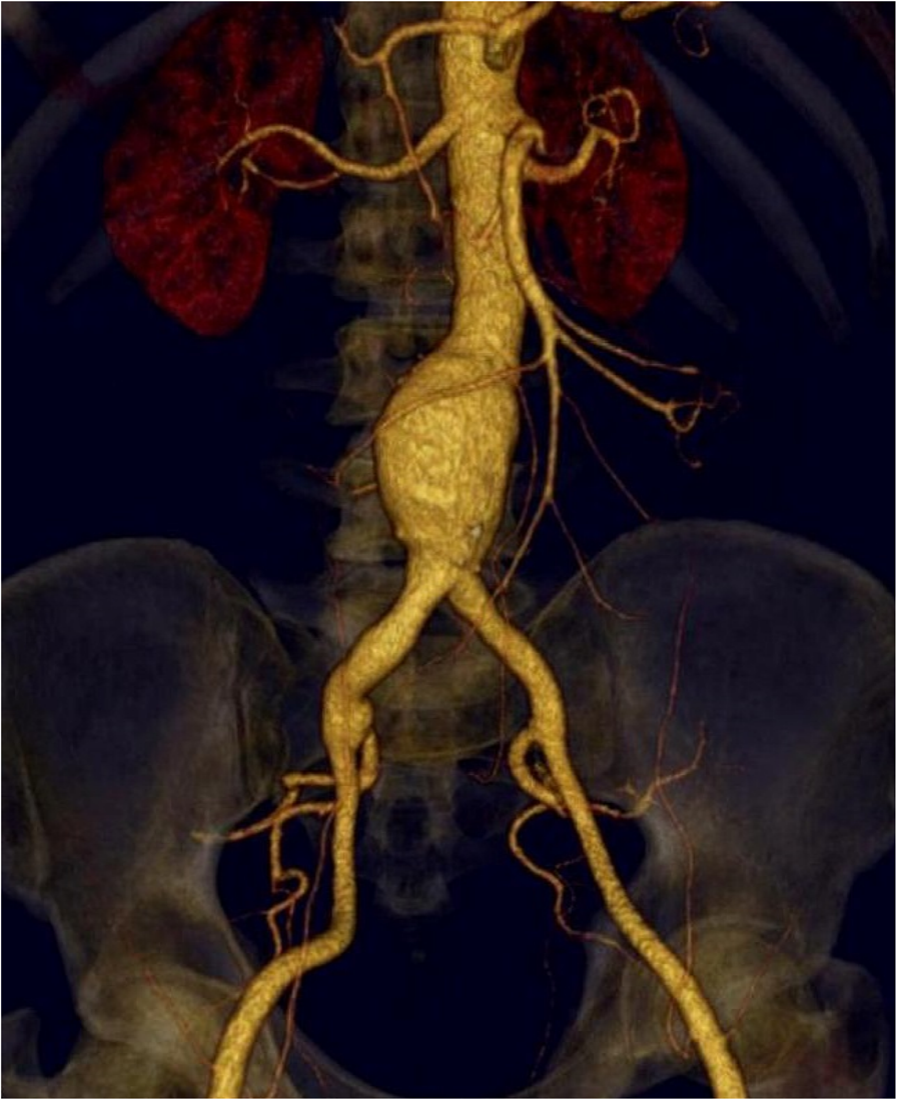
CTA showing infrarenal abdominal aortic and iliac aneurysms
